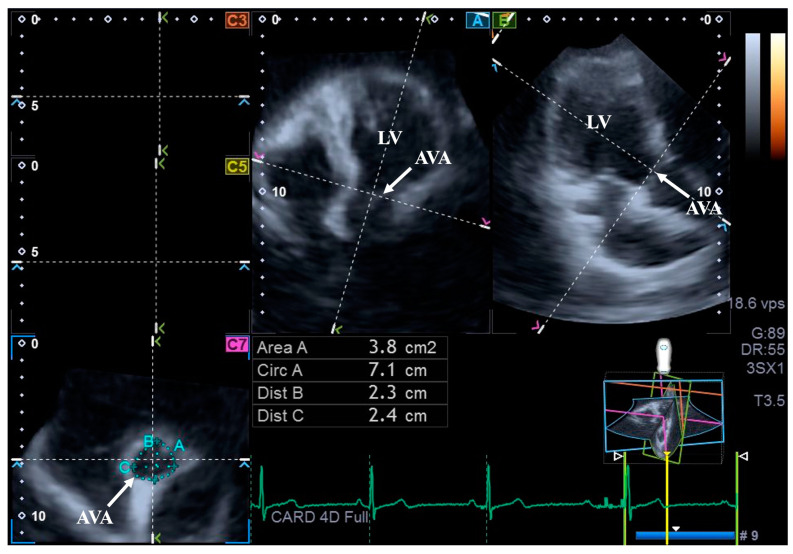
Assessment of the aortic valve annular dimensions by three-dimensional speckle-tracking echocardiography. Abbreviations: LV = left ventricle; AVA = aortic valve annulus; Area = AVA area; Circ = AVA perimeter; Dist B = maximum AVA diameter; Dist C = minimum AVA diameter.
